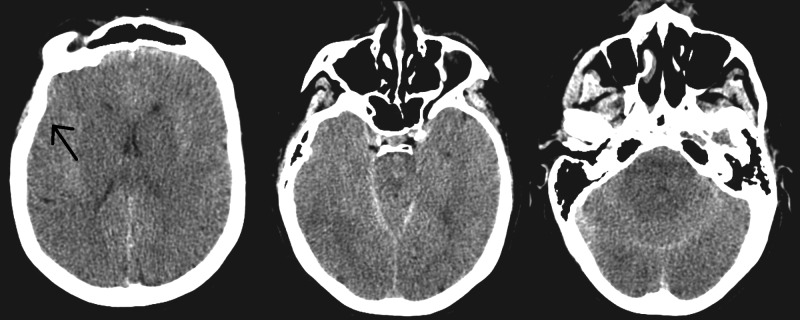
Postoperative day zero computed tomography of the head Diffuse cerebral edema, obliteration of cerebral of sulci and gyri is seen, consistent with an anoxic brain injury
